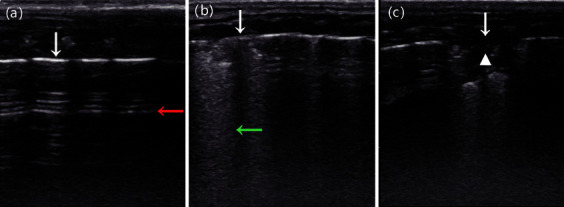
(a) Lung ultrasound score 0, the pleural line (white arrow) and A-line (red arrow) are clear. (b) Lung ultrasound score 1, the pleural line (white arrow) and A-line are blurred or disappeared, and B-line (green arrow) can be seen. (c) Lung ultrasound score 2, the pleural line (white arrow) disappeared with lung consolidation and air bronchogram (white triangle area).
